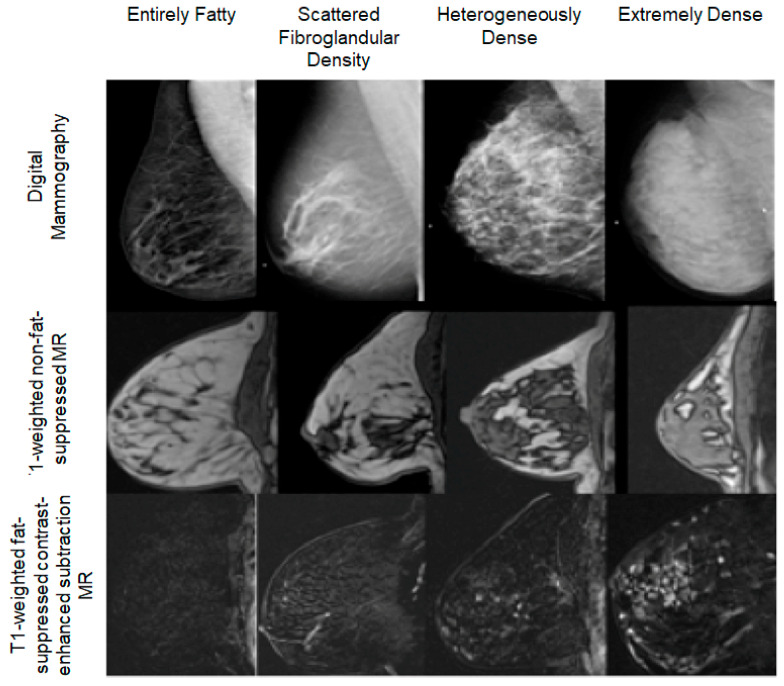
Imaging appearance of different breasts of increasing density using three types of imaging modalities. Digital mammography depicts how breasts appear more opaque as breast density increases. T1-weighted non-fat-suppressed MR images show increasing amount of fibroglandular tissue (FGT). T1-weighted fat-suppressed contrast-enhanced subtraction MR images show increasing amounts of background parenchymal enhancement (BPE), which reflects the vascularity of the fibroglandular tissue. (Adapted from [23]).
